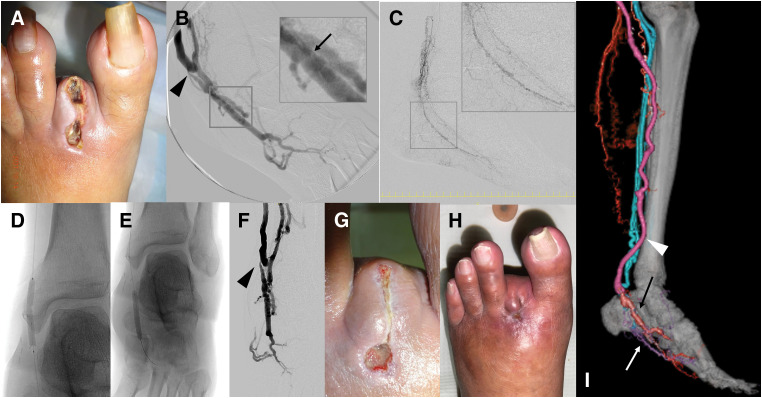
Postoperative course and intervention following surgical DVA. At 2 weeks postoperatively, the stump of the left 2nd toe exhibited no evidence of epithelialization; however, local infection remained well controlled (A). Angiographic evaluation revealed a focal stenosis at the DVA anastomotic site (arrowhead) and a suspected venous valve (arrow) impeding retrograde flow (B). At 1 month, endovascular reintervention was undertaken. A microcatheter was advanced to the plantar artery anastomosis, and contrast injection delineated the plantar artery via the diseased venous segment (C). Balloon angioplasty was performed using a 3 mm balloon to address both the anastomotic narrowing and the valve-related stenosis (D, E). Despite only modest angiographic improvement at the anastomotic site (arrowhead), venous opacification via the DVA was markedly augmented, indicating effective distal perfusion (F). At 2 months, the toe stump demonstrated progressive reduction in size (G). Complete wound healing was achieved by 3 months postoperatively (H). One-year follow-up CTA confirmed sustained patency of the vein graft (arrowhead), with clear delineation of the DVA (black arrow) and the plantar artery (white arrow) (I). DVA, distal venous arterialization
